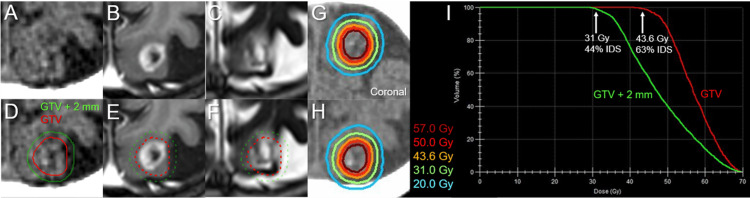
Target definition, planned dose distribution, and dose-volume histograms for the re-radiosurgery The images show CT findings (A, D, G, H); CE-T1-WIs (B, E); T2-WIs (C, F); target definitions (D, E, F); dose distributions (G, H); axial views (A-F, H); a coronal view (G); and dose-volume histograms (I) (A-F) These images are shown at the same magnification and coordinates under co-registration and fusions. (D-F) The contours of the defined GTV and the GTV + 2 mm structure are superimposed onto each image, where the GTV is intentionally oversized to include the surrounding brain surface. (G, H) Five representative isodose lines are superimposed onto CT images. (I) The arrows indicate the coverage of the GTV and GTV + 2 mm with 43.6 Gy and 31.0 Gy, respectively GTV: gross tumor volume; IDS: isodose surface; CT: computed tomography; CE: contrast-enhanced; WIs: weighted images
